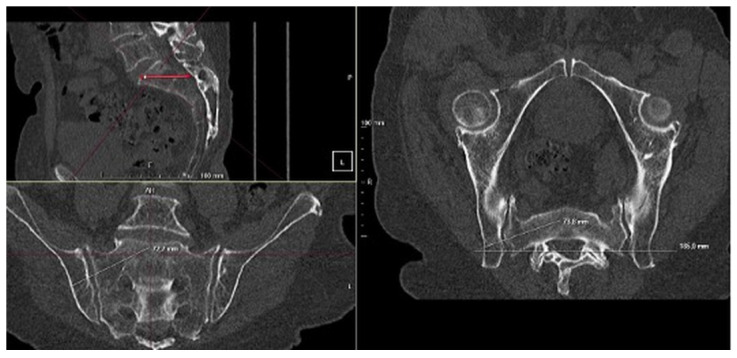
Preoperative CT-based planning and corridor measurement for the modified S1 trajectory. Multiplanar CT reconstructions (sagittal, coronal, and axial) are used to define the modified S1 corridor and determine the planned implant trajectory and length. The preoperatively measured implant length serves as an important intraoperative reference. In our practice, a discrepancy of approximately one implant-length increment, i.e., ≥5 mm from the planned length, prompts reassessment of the trajectory using lateral, inlet, and outlet fluoroscopic views, and intraoperative 3D imaging where available. A substantially shorter measured length may indicate that the trajectory is too steep, with risk of ventral sacral exit and potential L5 nerve conflict, whereas a substantially longer measured length may indicate that the trajectory is too flat, with risk of S1 neuroforaminal breach.
